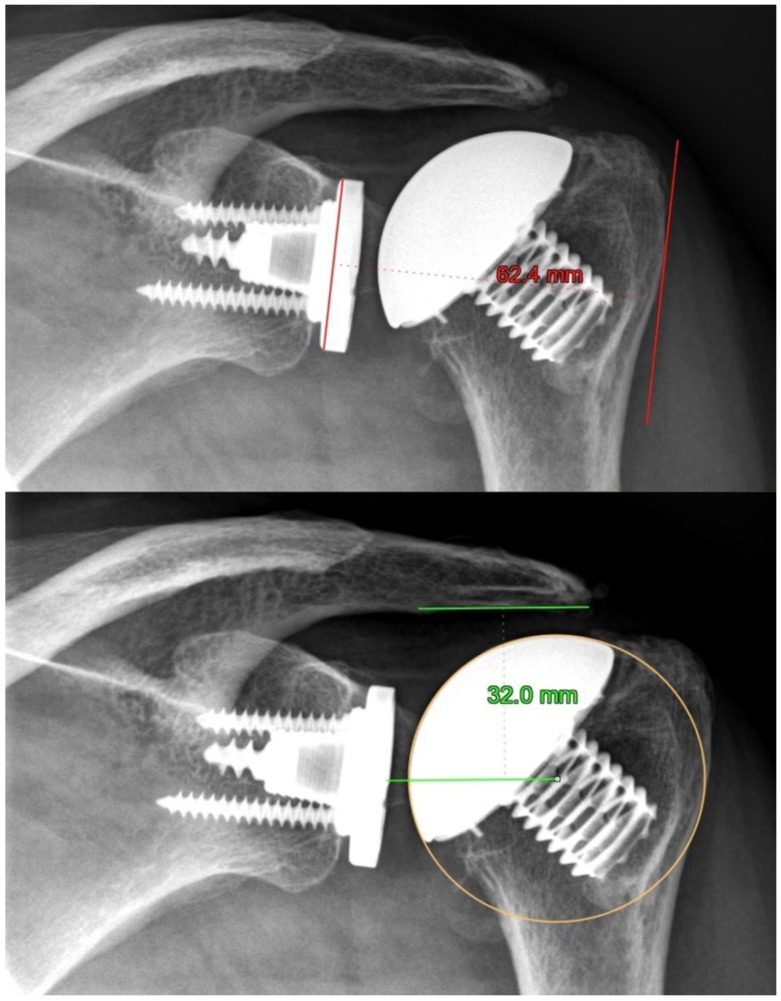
Postoperative radiographs of a left shoulder demonstrate the measurement method of the lateral glenohumeral offset (above X-ray image) and the humeral head migration (below X-ray image).
